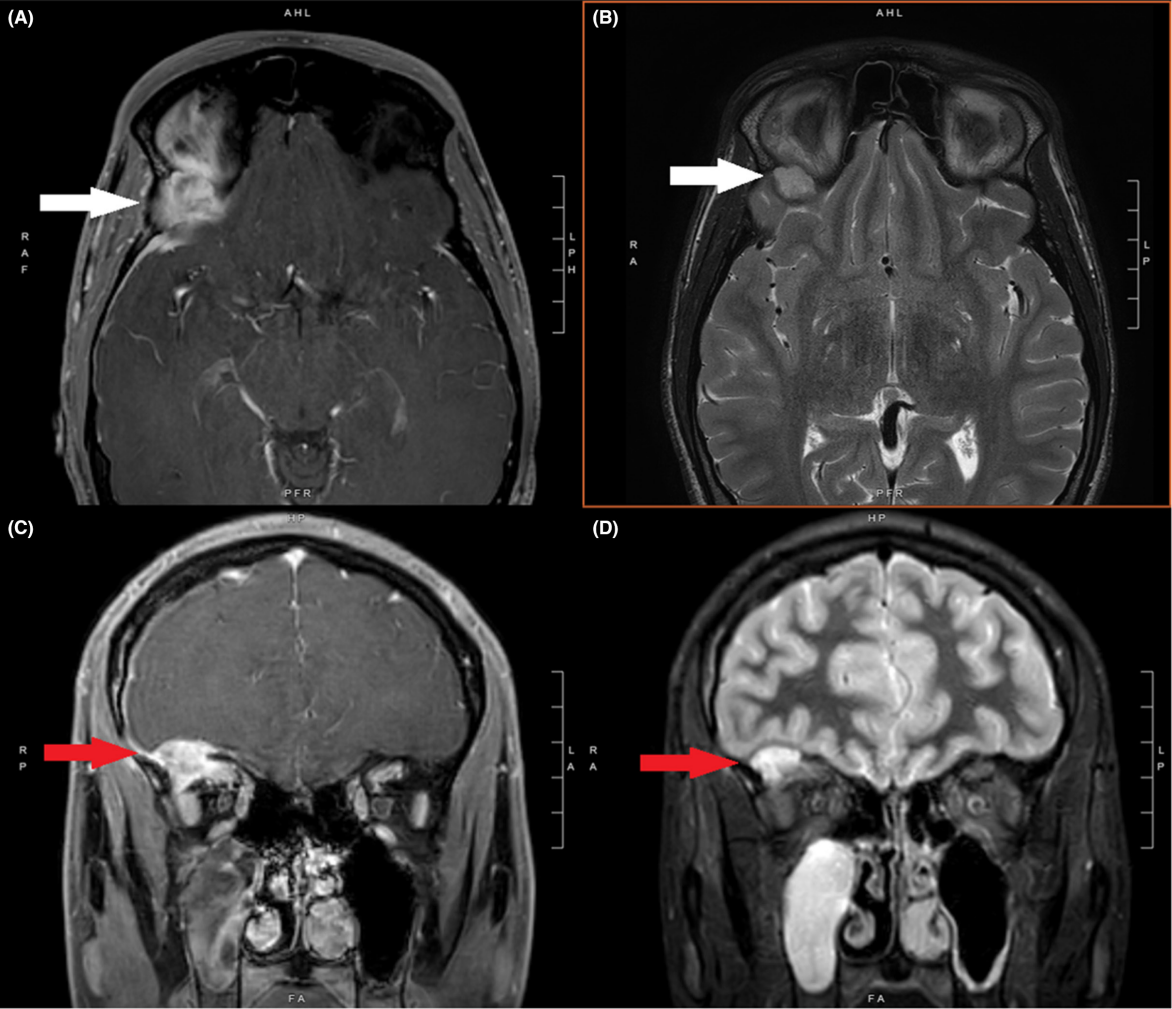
Initial MRI of the brain demonstrating enhancing heterogeneous mass (arrow) in the superior‐lateral aspect of the right orbital roof and extension into the anterior cranial fossa. (A) T1 post‐contrast axial (B) T2 axial (C) T1 post‐contrast coronal (D) T2 coronal.
